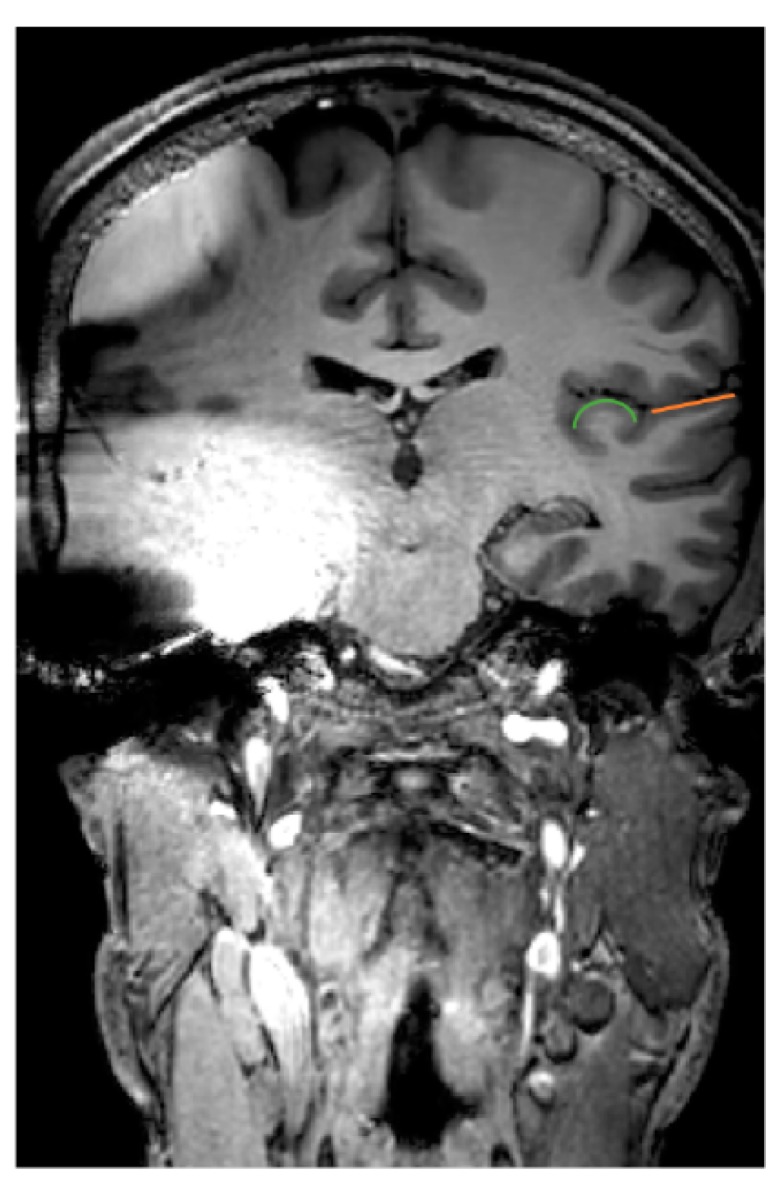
MRI artefacts caused by a unilateral CI on a coronal slice at the level of the auditory cortex. On the hemisphere contralateral to the device, the primary auditory cortex (Heschl’s gyrus) is depicted in *green* and the secondary auditory cortex (planum temporale) in *orange*.
